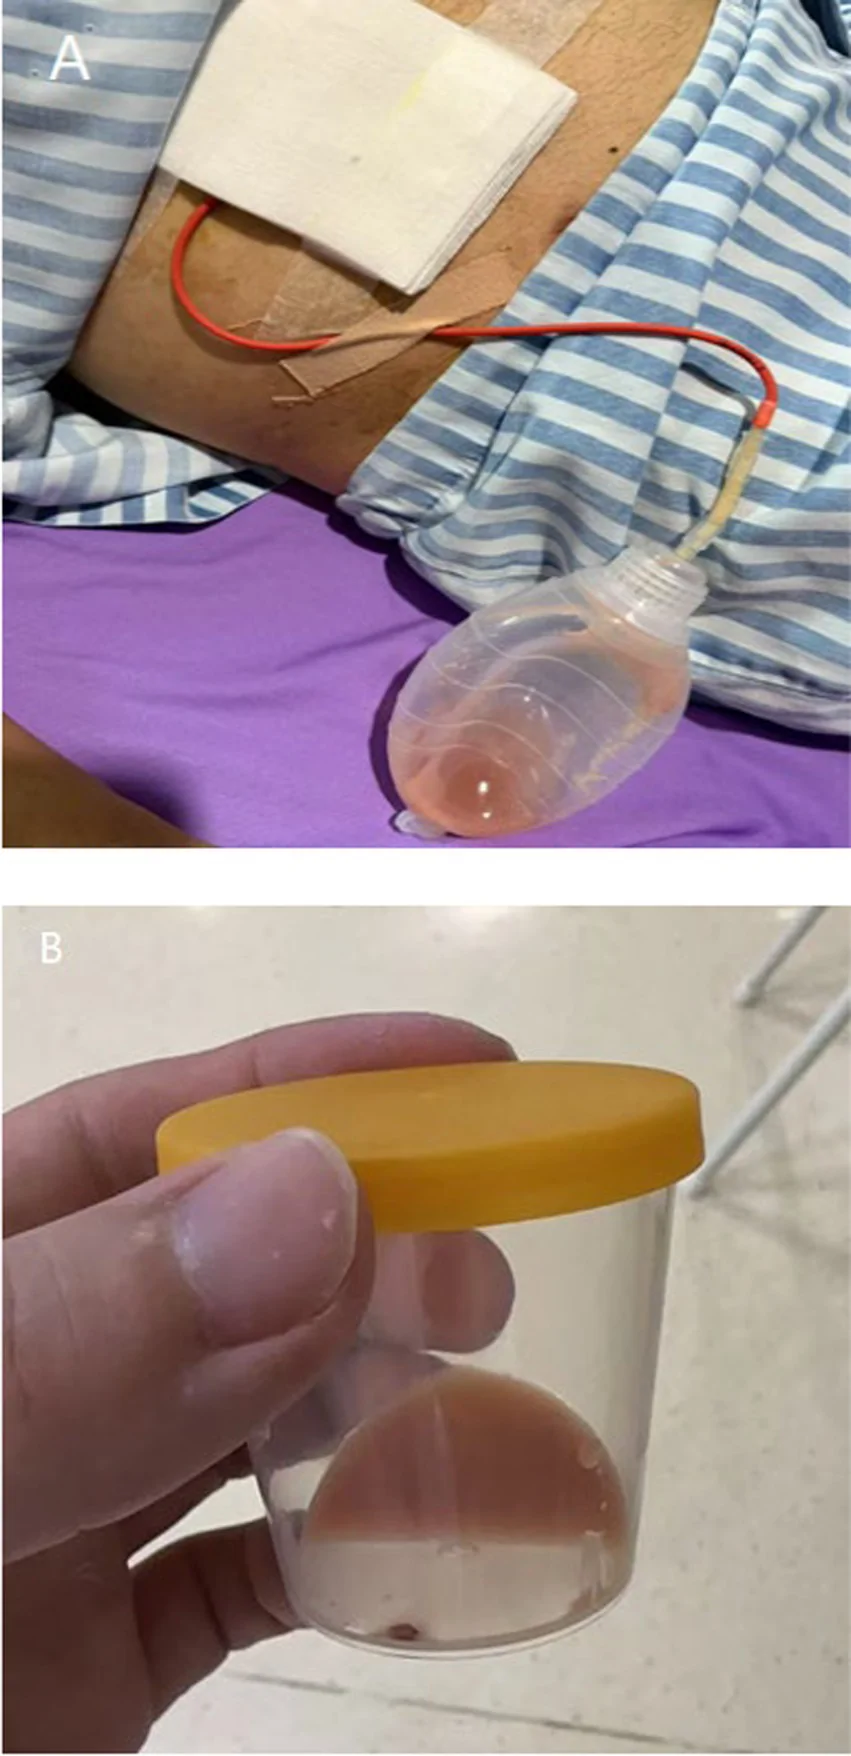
Ultrasound-guided source control on HD31. An 8 French (8Fr) drainage catheter was inserted through the original sinus tract and connected to a low-negative-pressure bulb, yielding thick dark-yellow fluid.
